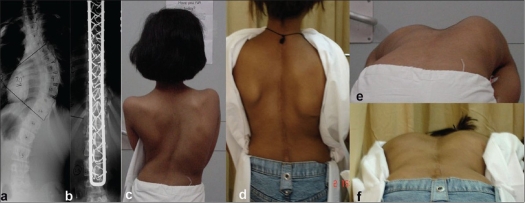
(a,b) Preoperative and post operative dorso lumbar x-rays in a girl with Turner's syndrome shows a good correction of scoliotic deformity. Clinical photographs (c-f) showing good correction and maintenance, alongwith rib hump improvement at 3 ½ years follow up
